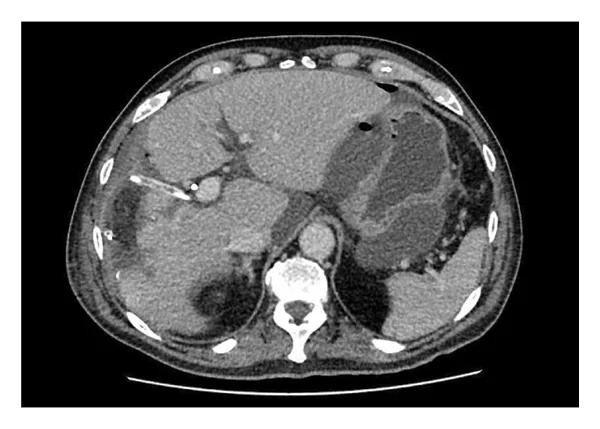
Postoperative appearance of multiloculated hepatic abscesses associated with *Enterococcus faecium* infection, with right hepatic ductal system stenting visualized during the portal phase of contrast‐enhanced abdominal CT.
